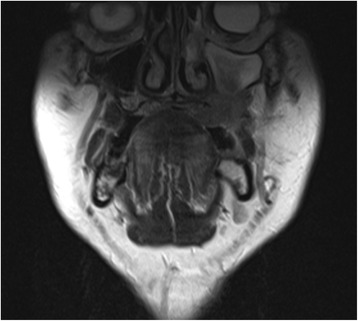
Staging MRI scan showing destructive lesion left maxilla
